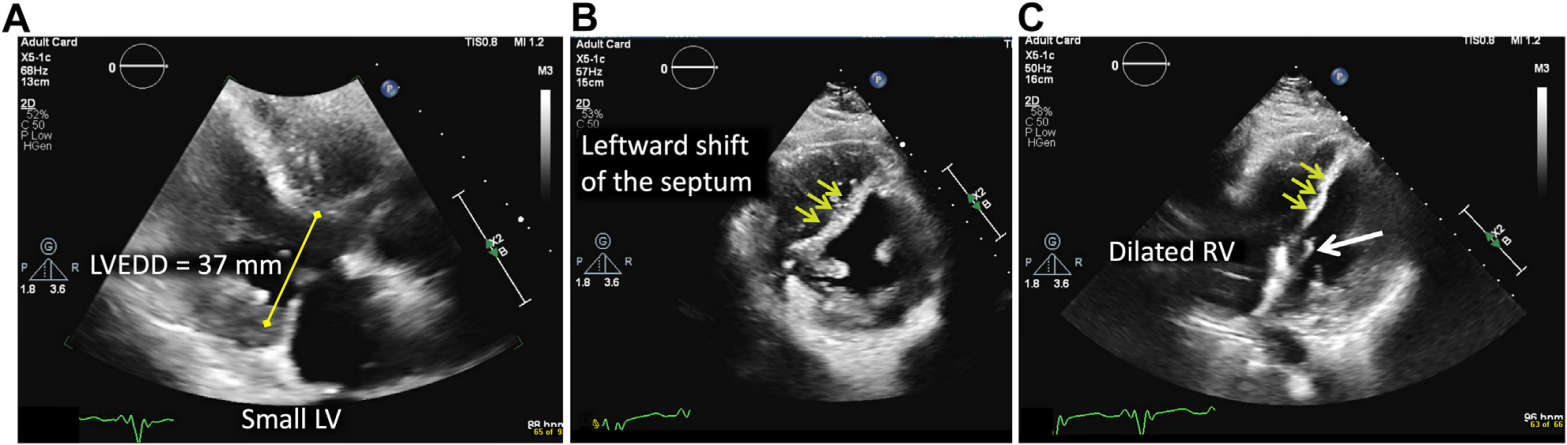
Two-dimensional TTE following percutaneous drainage of the MFC, parasternal long-axis (A), short-axis (B), and apical four-chamber (C) diastolic views, demonstrates a smaller left ventricular cavity (left ventricular end-diastolic diameter [LVEED], 37 mm), new leftward position of the IVS *(yellow arrows)*, and new dilation of the RV; also seen is tethering of the mitral valve leaflets *(white arrow)*. *LV*, Left ventricle.
